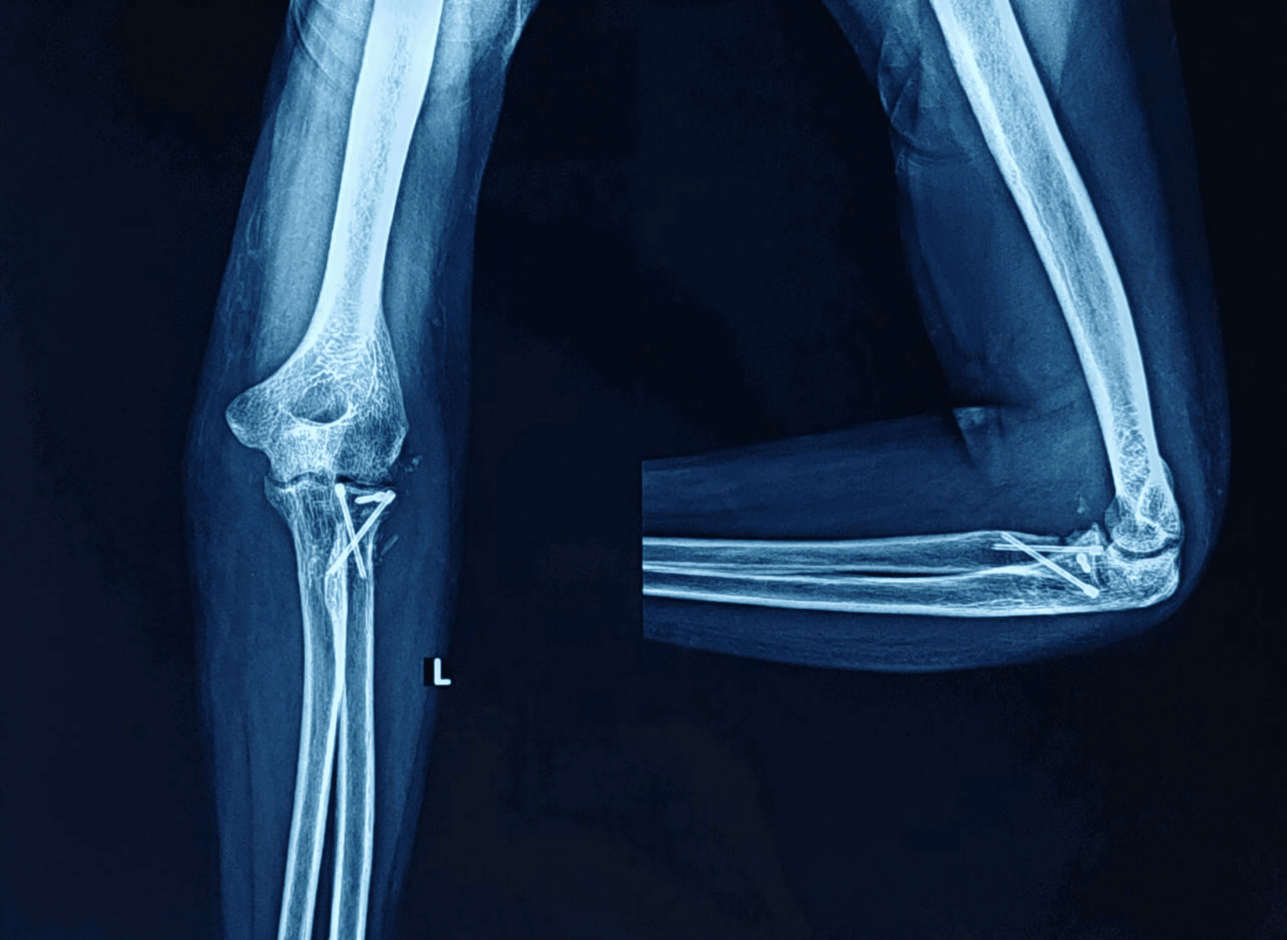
One-month follow-up X-ray depicting AP and lateral views of the left elbow joint AP: Anteroposterior
